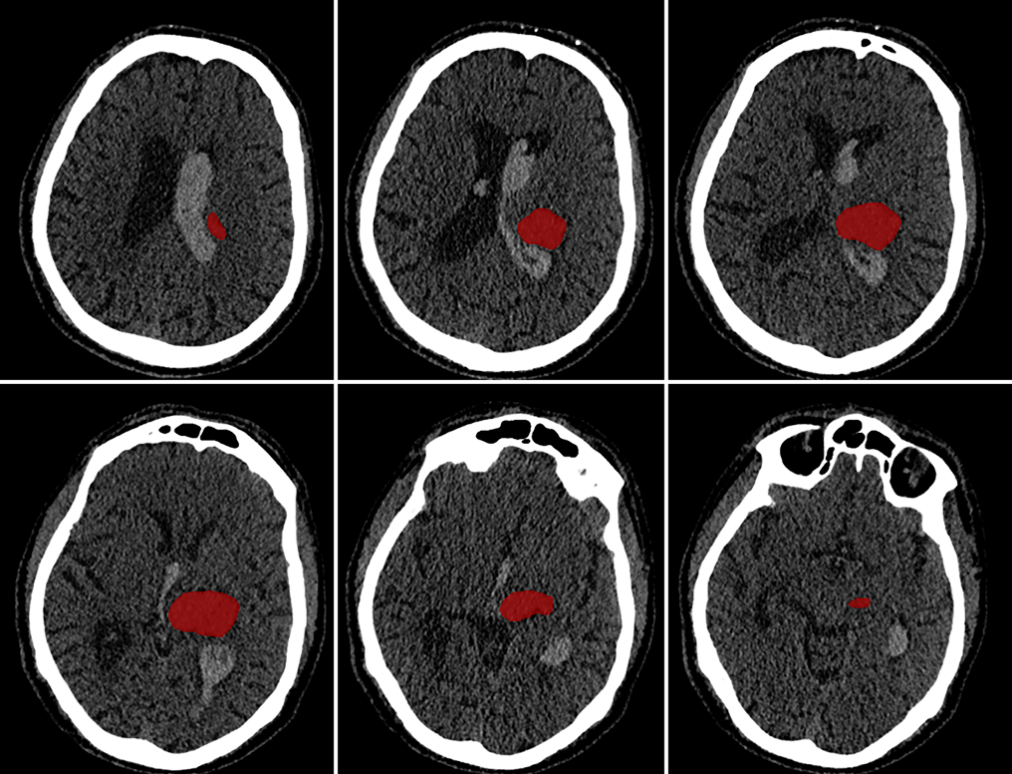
CT scan of a patient with ICH and intraventricular extension. Lesion segmentation of intraparenchymal blood performed using the nnU-Net 3D full-resolution network trained in the context of this study
